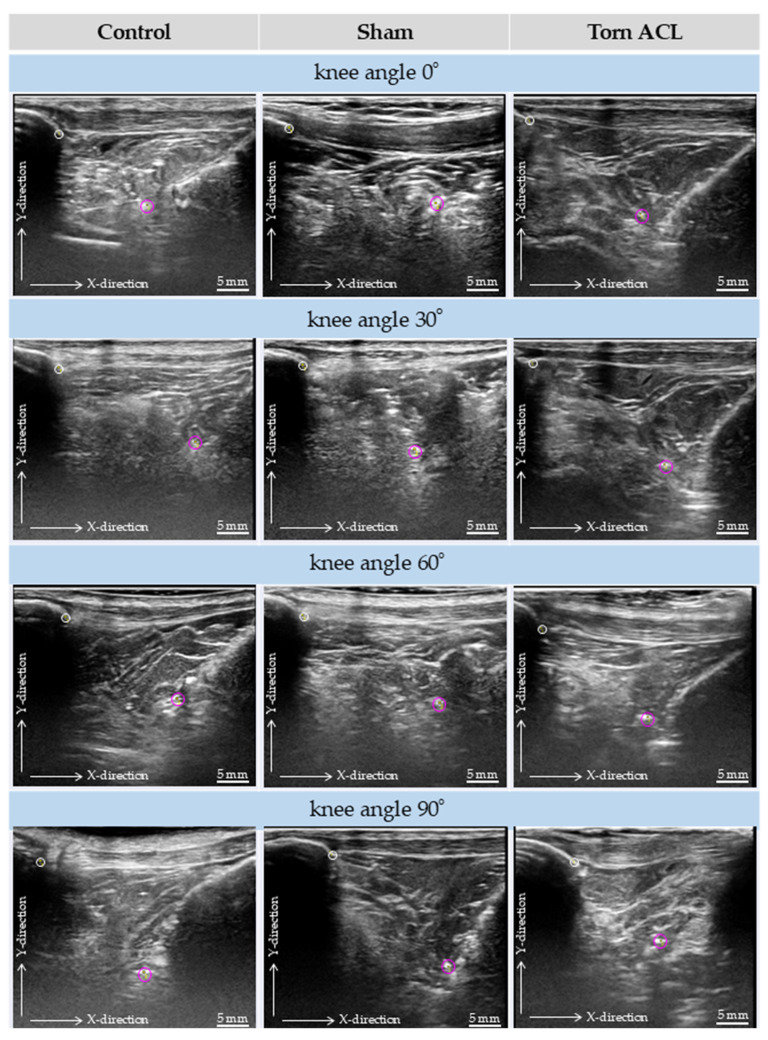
Long-axis ultrasound images of the IPFP in the three groups, visualized at the four set flexion angles. The metallic clip (purple circle) is inserted into the AFC-SEG, and the anchoring point is indicated by the white circle.
